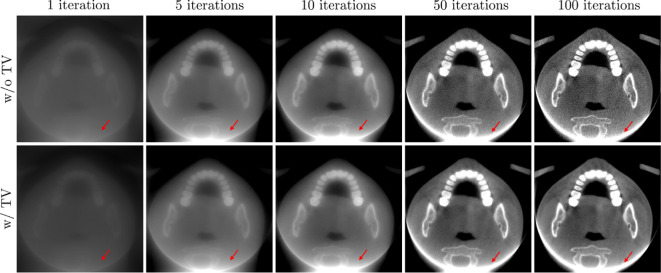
Results of the iterative reconstruction model in (3.1) over iterations for three-dimensional dental CBCT with a truncated FOV. The first and second rows present the results with and without total variation regularization, respectively. As iterations progress, the discrepancy between the actual projection and the forward projection within the truncated FOV accumulates regardless of regularization, leading to significant degradation in the reconstructed CT image quality, as indicated by the red arrows.
